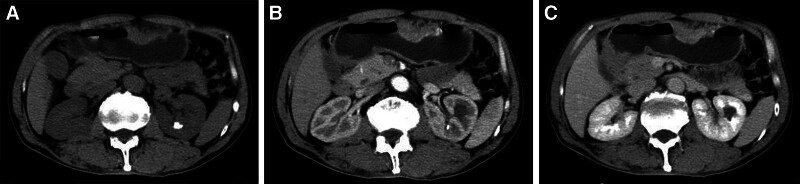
A typical case (male, 64 years old, microvascular invasion (+)) with local gastric wall thickening with abnormal enhancement on CT (T3 stage, N3 stage, adenocarcinoma, nonpoor differentiated, diffuse type). (A) Plain CT image: local gastric wall thickening in the gastric body. (B and C) Enhanced CT image: the thickened gastric wall in the gastric body is significantly enhanced.
